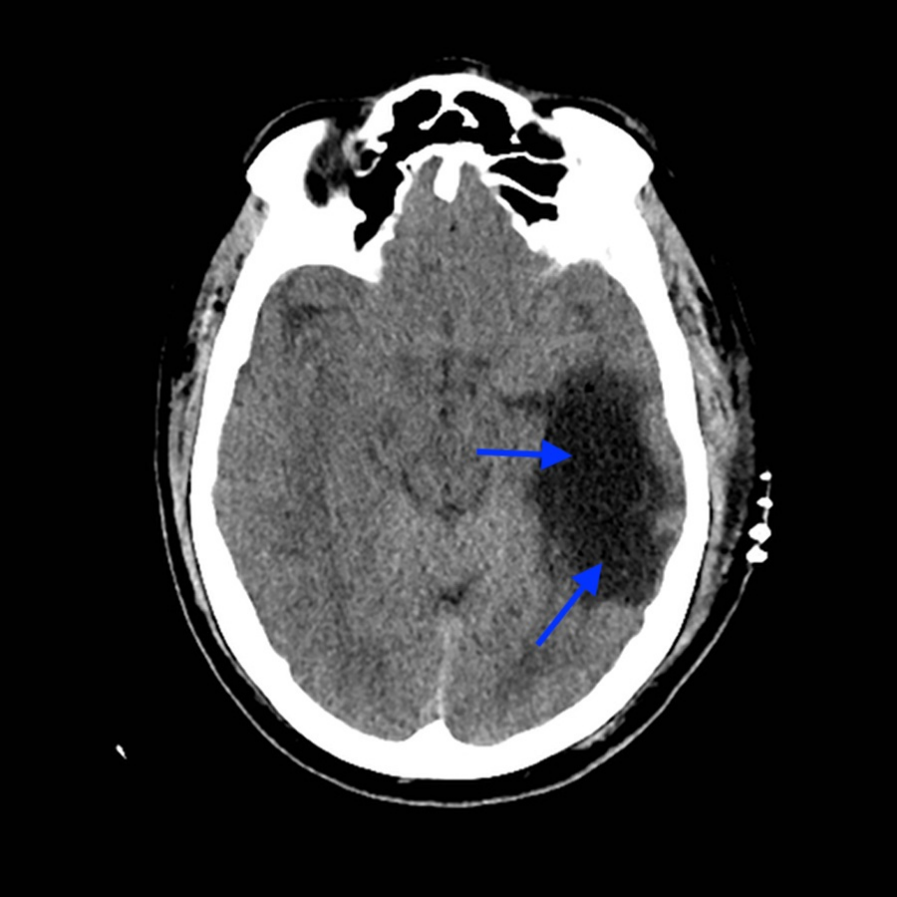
Axial unenhanced CT of the head with large lucency (blue arrows) in the left temporal lobe. CT - computed tomography
